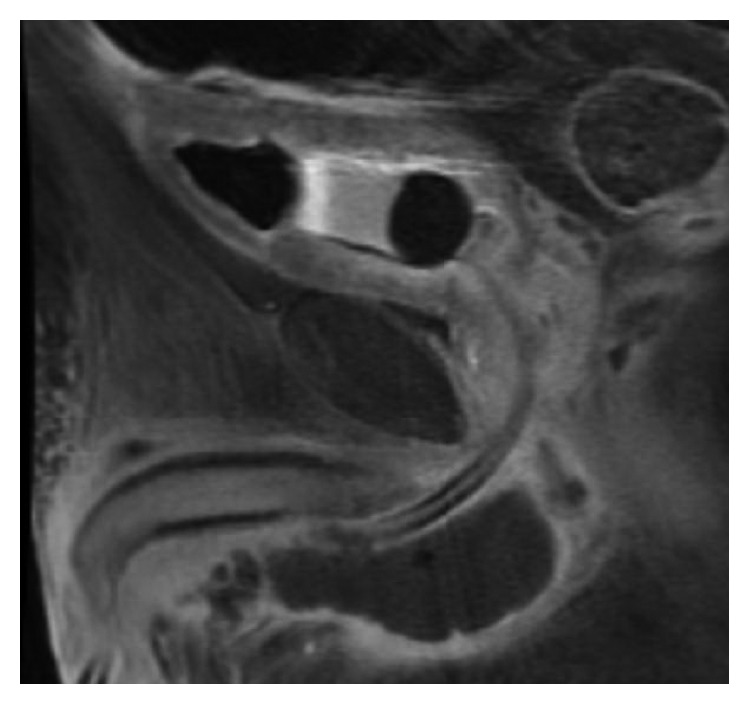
Sagittal view on MRI T1 image with gadolinium demonstrating decreased signal intensity in the corpus spongiosum compared to the corpora cavernosa consistent with reduced blood flow.
